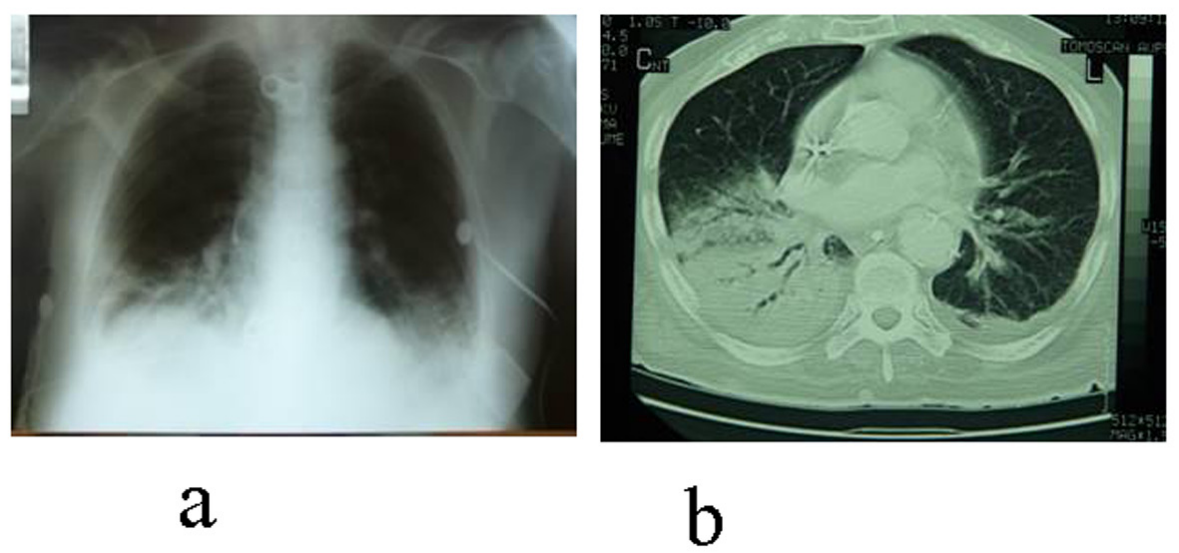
Chest X-ray (a) and CT (b) reveal pneumonia of the right basis with accompanying plevritis.
